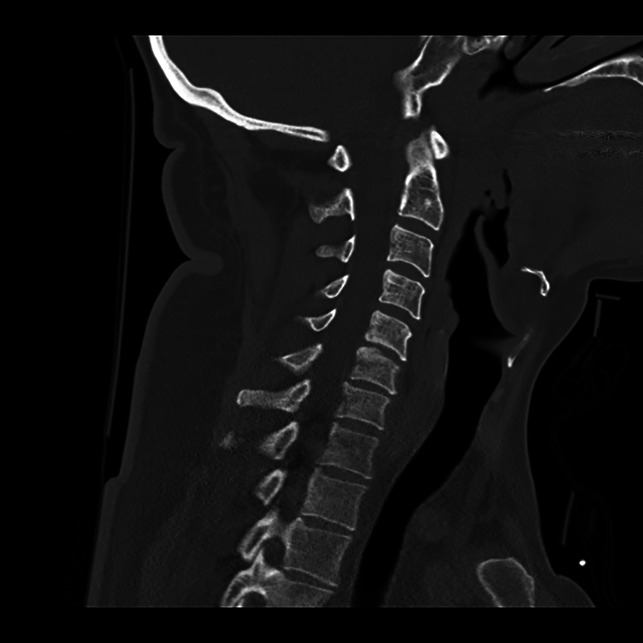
Radiograph showing undisplaced right C6 facet fracture. Potential for instability AO SLIC (F2).
